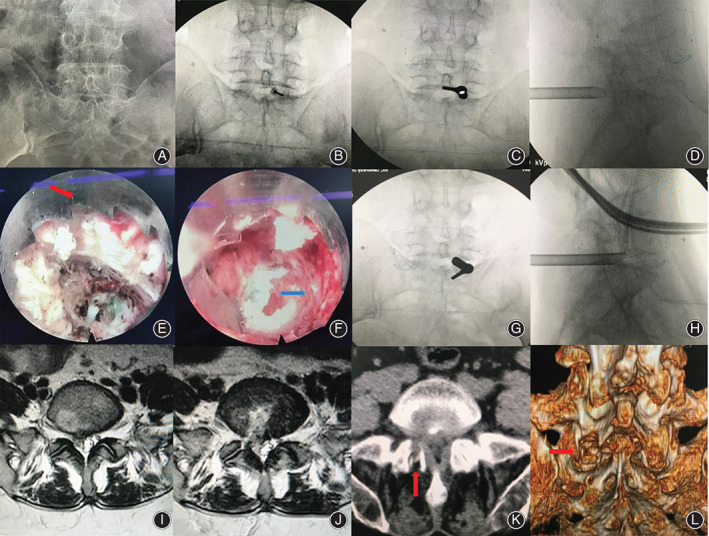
Typical case three. (A) Lumbar anterior–posterior X‐ray image. Narrow interlaminar window of L_5_–S_1_ level. (B, C, and D) The target puncture point and working cannula were located at the safety zone. (E and F) Endoscopic visible trephine (red arrow) was used for the laminoplasty (blue arrow). (G and H) Working cannula reached herniated disc tissue *via* the spinal canal under endoscopic observation. (I and J) Preoperative and postoperative MRI showed removal and good decompression of the S_1_ nerve root and dura. (K and L) Postoperative CT scan revealed laminoplasty (arrow).
